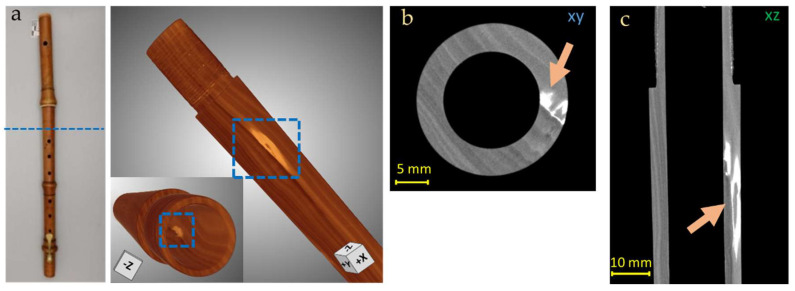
Original and 3D render (a) and CT horizontal (b) and vertical (c) slices of the Transverse Flute body (left hand), in which area of higher density was observed (orange arrows), also visible in the 3D model (blue squares). Blue line in the original pictures indicates the high of descripted feature.
